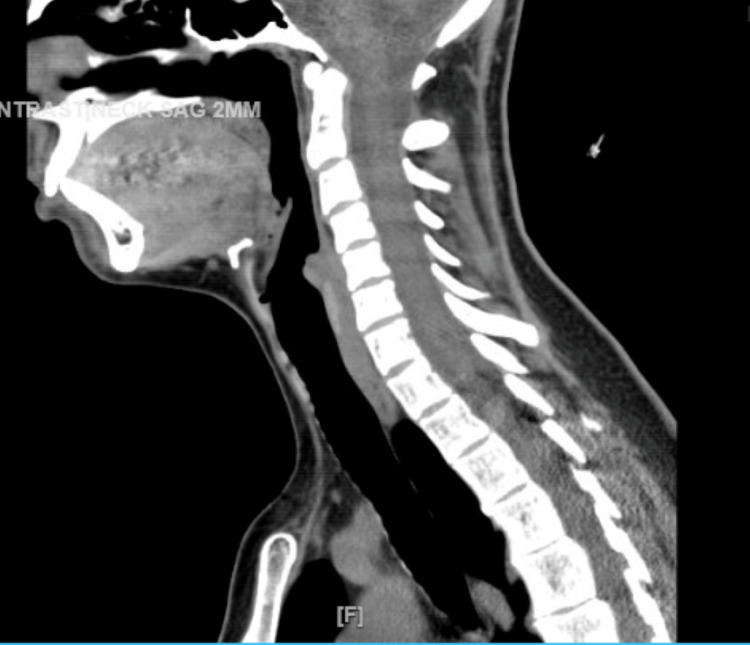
A CT scan of the soft tissues of the neck (without contrast) during discharge from the medical floor
